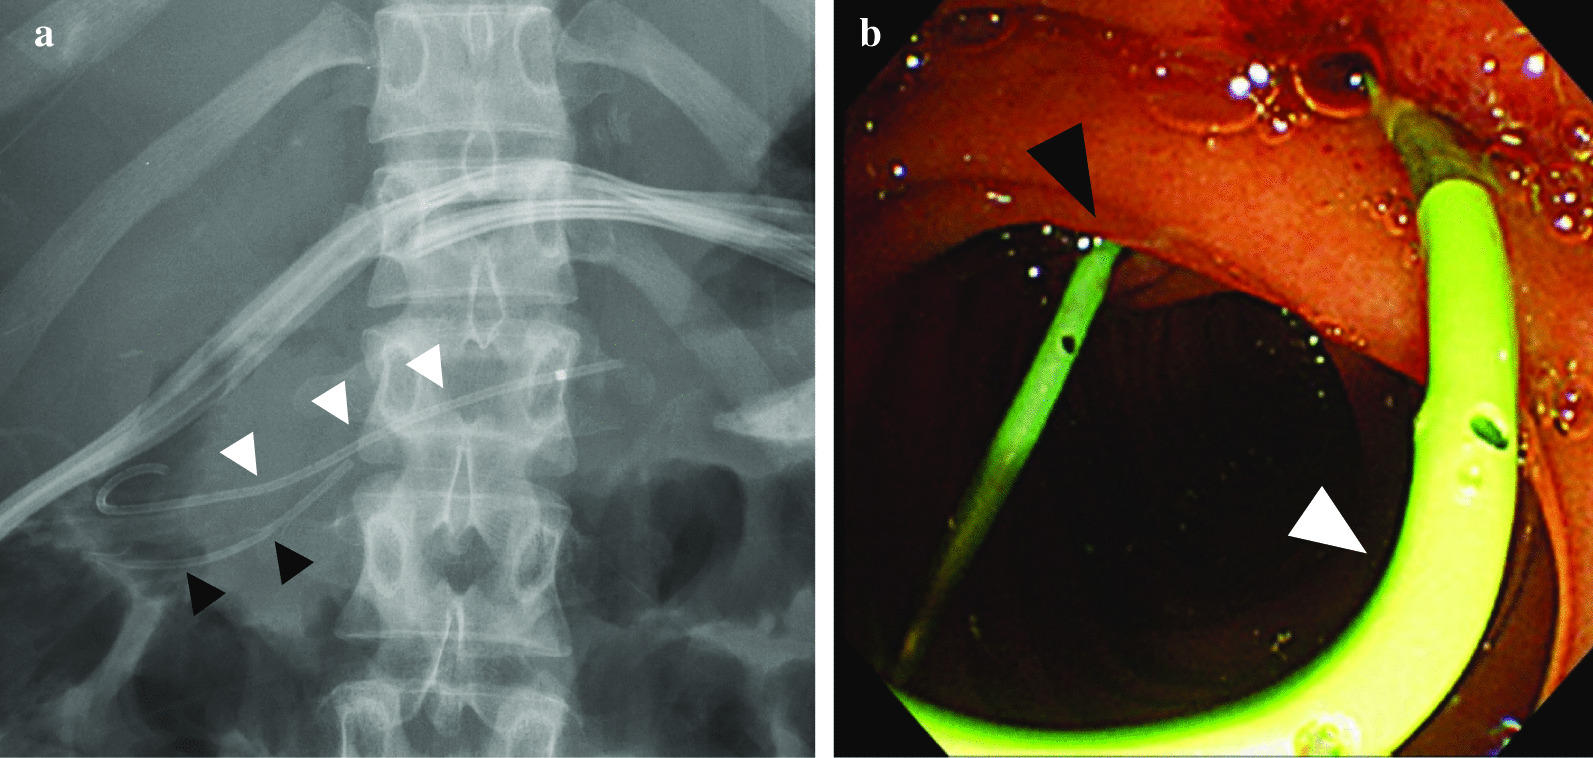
Abdominal X-ray and endoscopic findings on day 9. The double pancreatic duct stenting is shown on abdominal X-ray (a) and endoscopic findings (b). The ENPD tube was replaced by a 5 Fr endoscopic pancreatic stenting (EPS) (white arrowheads) for internal drainage on day 9. The black arrowheads show the ERPD tube that was placed on day 3
